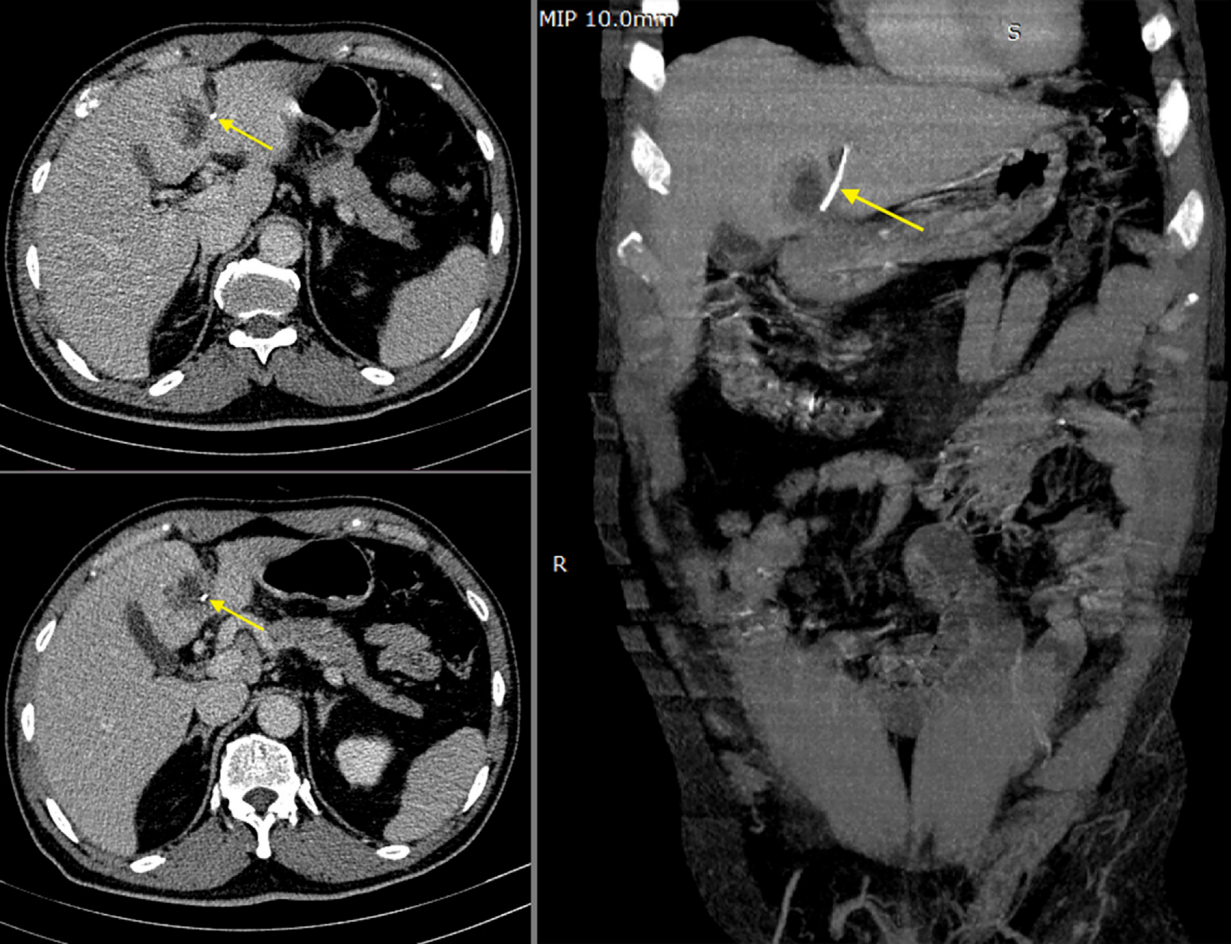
CT scan of the abdomen showed an abscess with a linear foreign body (arrow) in segment 4B and segment 3 of the liver.
